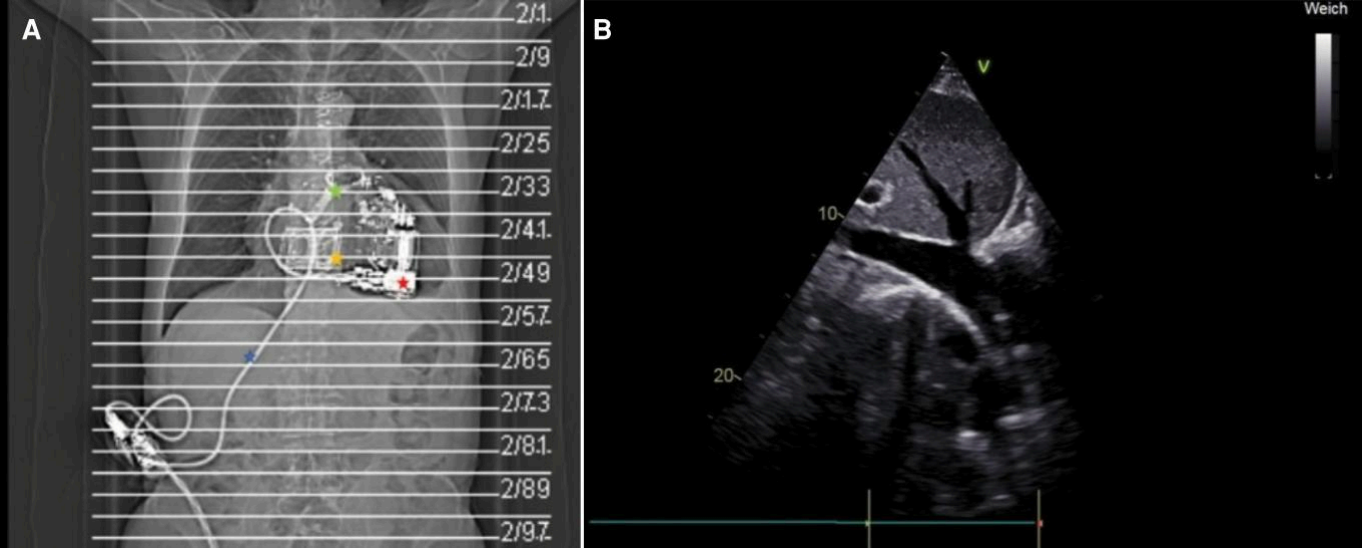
(*A*) Computed tomography of the Aeson® CARMAT Total Artificial Heart device. The red star marks the main part of the prosthesis, which contains two micropumps, embedded electronics, and sensors. The yellow star indicates the connection between the atrial flanges and the atrial interface following ventricular removal. The green star marks the pulmonary and aortic valves. The blue star denotes the driveline. (*B*) Due to acoustic shadowing from the prosthesis, transthoracic echocardiography is limited, allowing only assessment of the inferior vena cava to estimate volume status.
